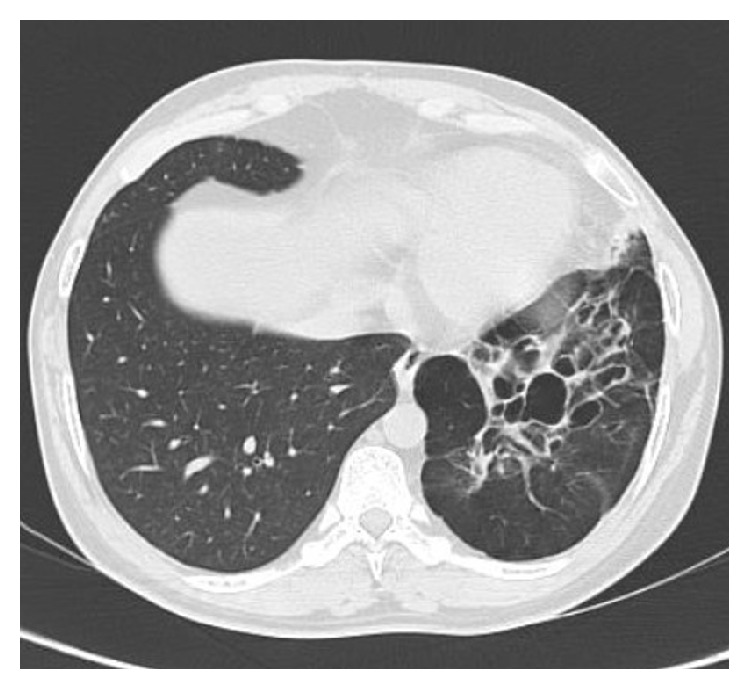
HRCT showing minimal bronchiectatic changes on the left side.
